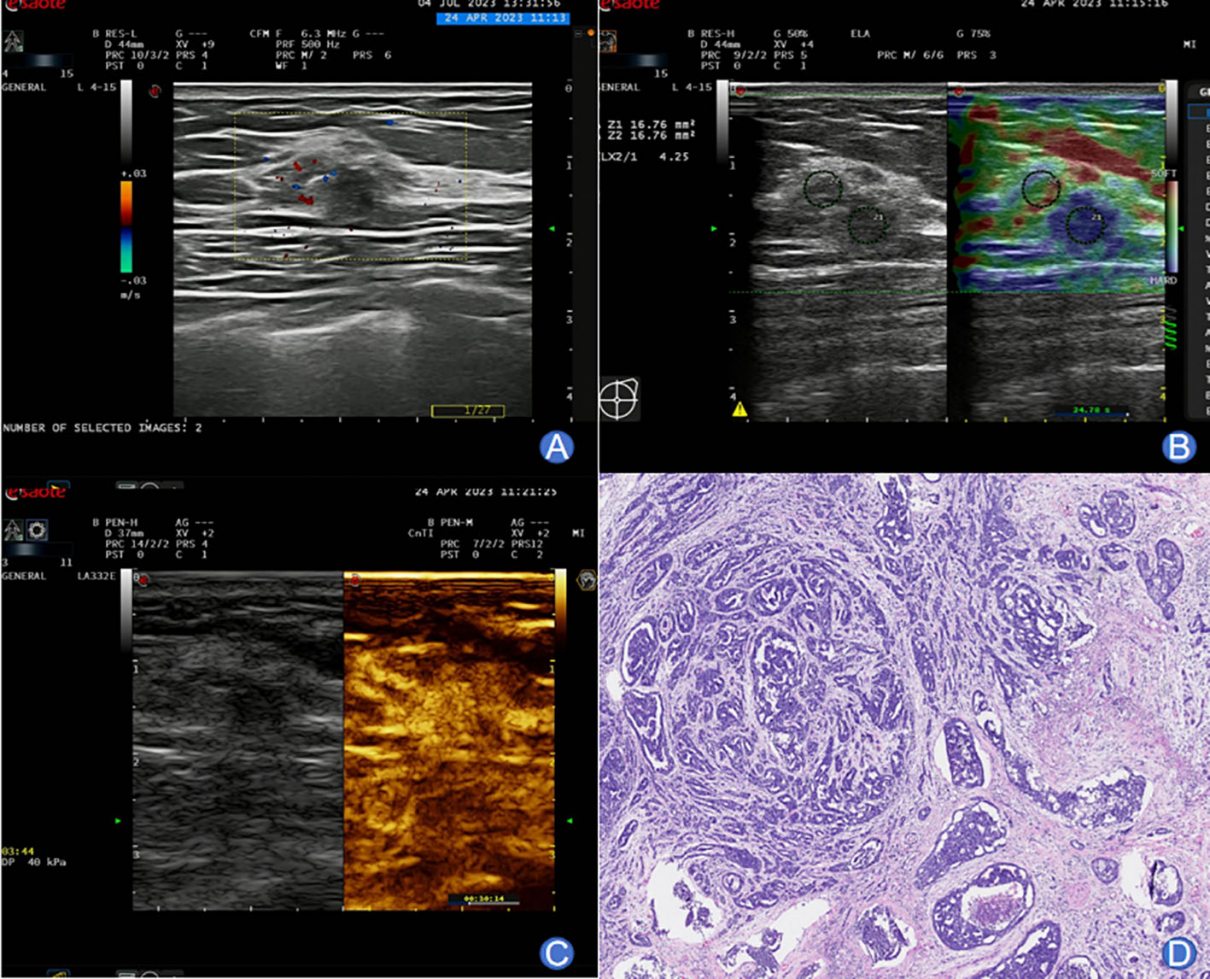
A 59-year-old female presenting typical images of malignant breast tumor. (A) Conventional ultrasound imaging revealed an irregularly shaped, partially indistinct, approximately 8×6.7 mm lesion within the right breast. The internal echoes were heterogeneous, and the surrounding tissue structure appeared distorted. Peripherally, blood flow signals were detected. (B) SWE imaging displayed a relatively stiff nodular texture, with an Emax value of 97 kPa. (C) Following a bolus injection of 4.8 mL SonoVue contrast agent, enhancement was observed at 13 seconds, reaching the peak at 28 seconds. The lesion exhibited centripetal high enhancement with the presence of large blood vessels entering. Enhancement extended beyond the range of 2D imaging, with an irregular morphology and unclear boundary. The washout occurred later than in the surrounding glandular tissues. (D) Pathological examination confirmed invasive ductal carcinoma of the non-special type.
